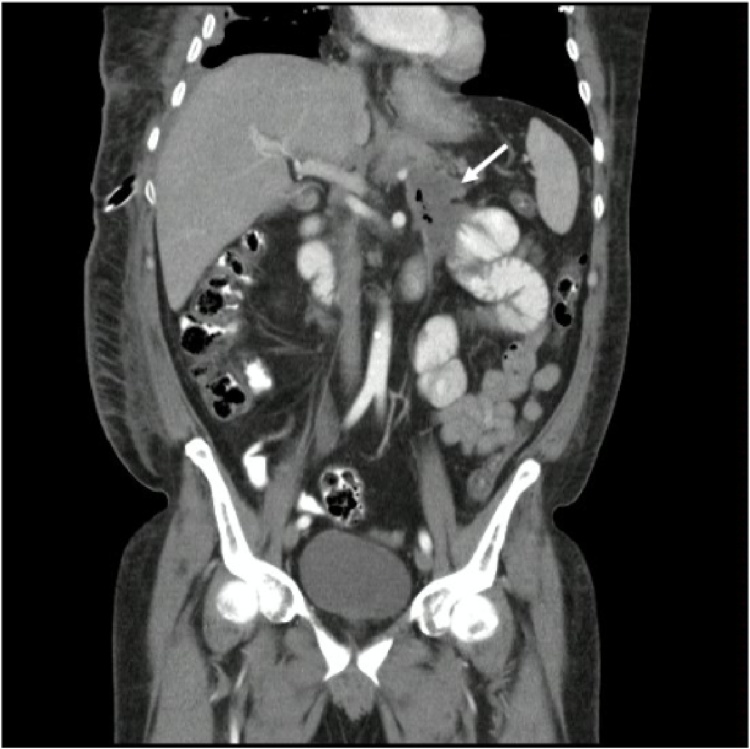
Double contrast Computed Tomography of the abdomen. Coronal reconstruction. The white arrow indicates the peripancreatic collection towards the tail of the pancreas.
